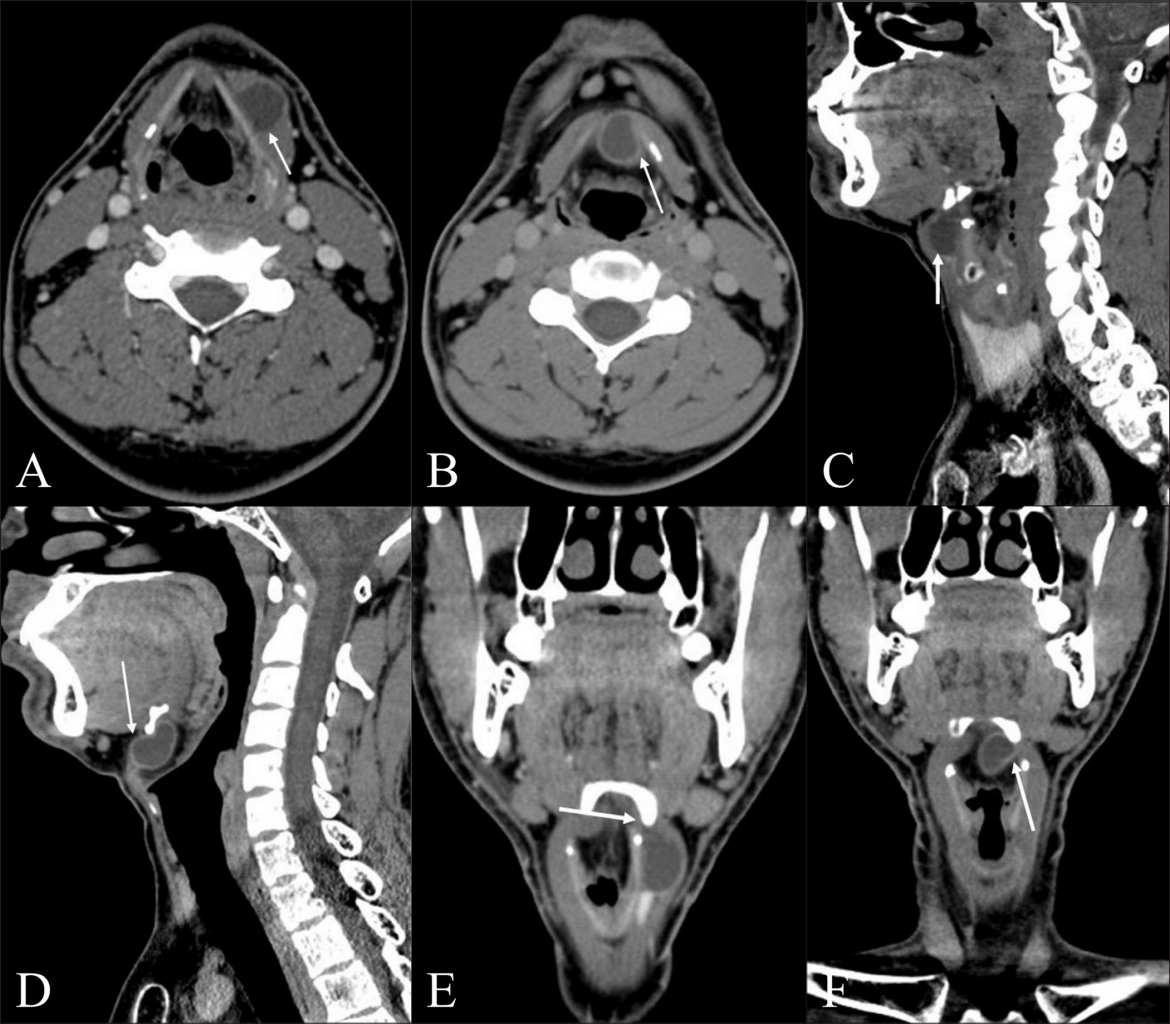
(A) Axial contrast-enhanced CT scans of the neck show a cystic, left paramedian lesion (arrow) located under the the strap muscles closely abutting the thyrohyoid membrane in the neutral position (B) The same lesion assuming an intralaryngeal location after hyperextension of the neck (C) Sagittal reformatted contrast-enhanced CT scans of the neck show a cystic, left paramedian lesion (arrow) located under the the strap muscles closely abutting the thyrohyoid membrane in the neutral position (D) The same lesion assuming an intralaryngeal location after hyperextension of the neck with probable defect in thyrohyoid membrane (E) Coronal reformatted contrast-enhanced CT scans of the neck show a cystic, left paramedian lesion (arrow) located under the the strap muscles closely abutting the thyrohyoid membrane in the neutral position (F) The same lesion assuming an intralaryngeal location after hyperextension of the neck with probable defect in thyrohyoid membrane
